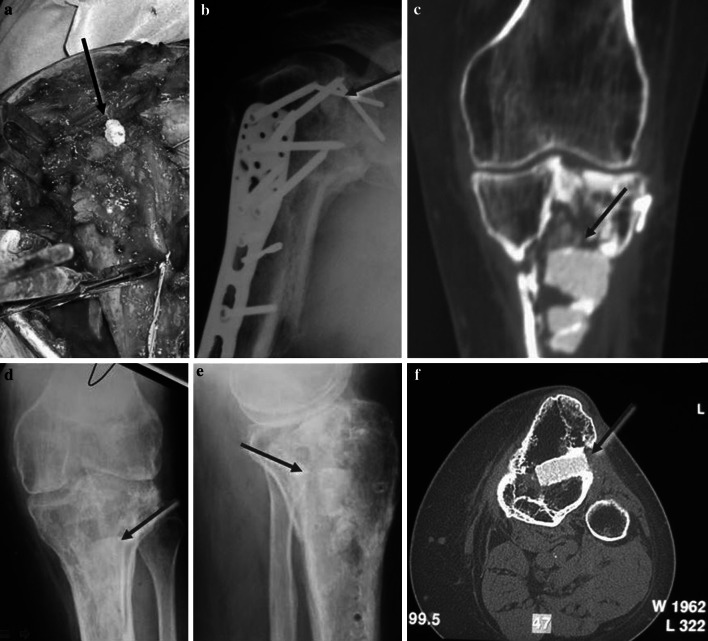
HA-TCP bone substitutes in proximal humeral and tibial traumatic bone loss. a Intraoperatory implant of the material in the proximal humerus. b X-ray control at 1 year show the substitute inside the humeral head. c–f X ray and CT scan at 3 year of follow up in the proximal tibia. The HA-TCP material resulted well osseointegrated, but without any sign of resorption or bone substitution
